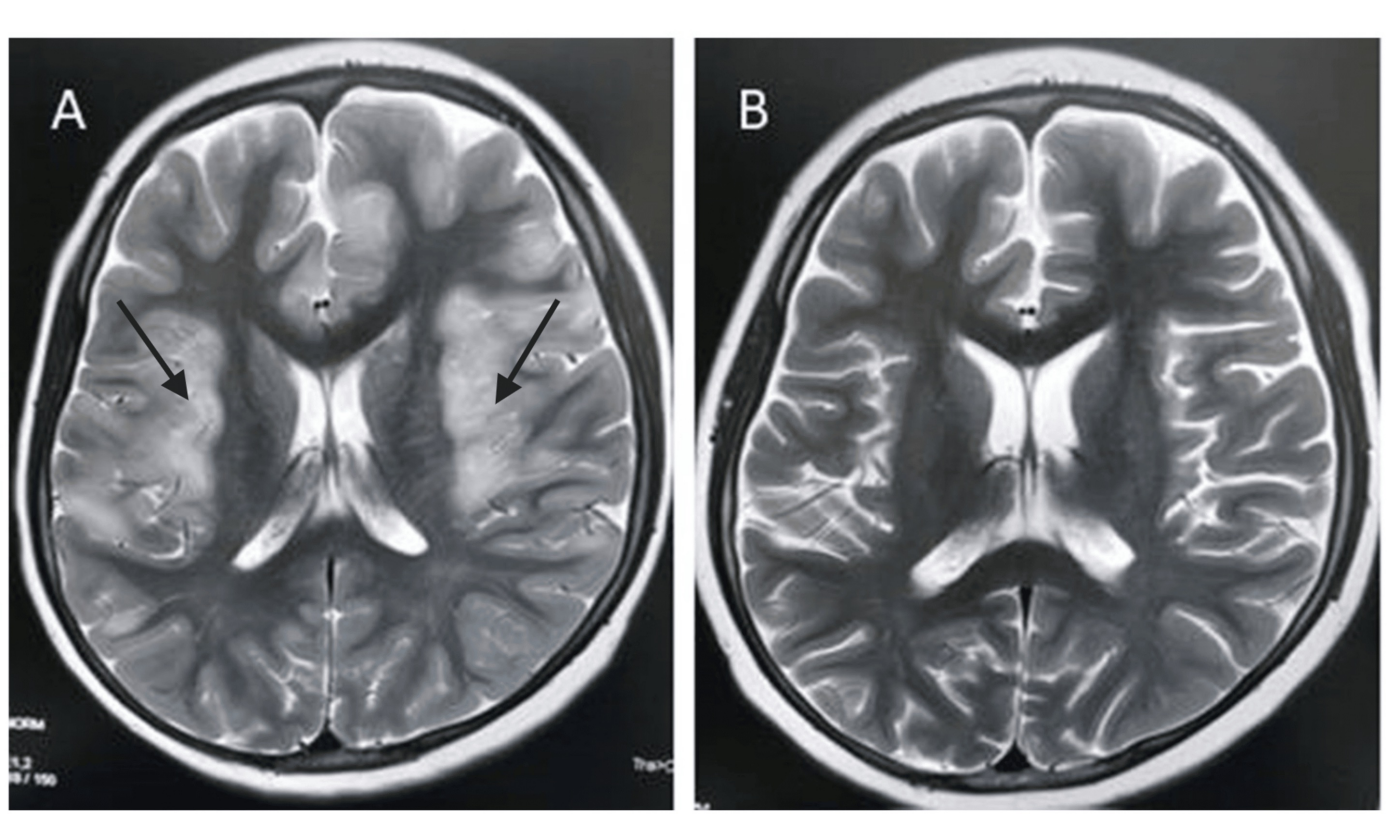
MRI brain showing the primary lesion and its resolution on follow-up (A) T2W axial image of the brain at the level of the lateral ventricles shows symmetric patchy juxta-cortical hyperintense signal involving the bilateral peri-insular white matter (black arrow); (B) T2W axial image corresponding to A, at the 6-week follow-up, shows complete resolution of the signal changes after steroid therapy. MRI: magnetic resonance imaging: T2W: T2-weighted
